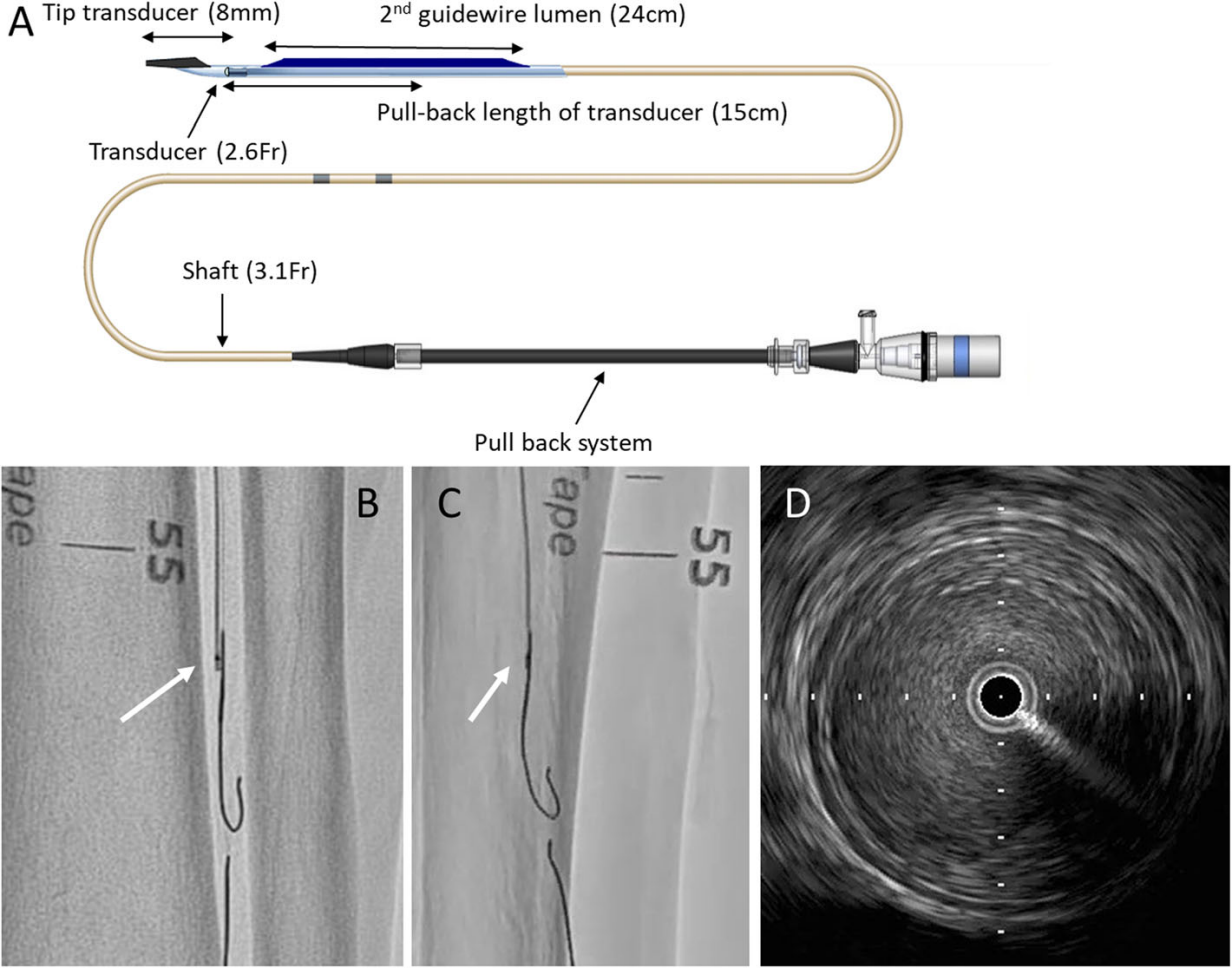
a Structure of AnteOwl WR (AnteOwl) intravascular ultrasound (IVUS). b, c Angiographic images of AnteOwl IVUS. b is the right anterior oblique view, and the transducer is to the left of the IVUS wire. Alternatively, c is the left anterior oblique view, and the transducer and IVUS wire are almost the same. The positional relationship between the transducer and IVUS wire can be understood in this way using rotational angiography. d Typical image of a superficial femoral artery lesion on AnteOwl IVUS
